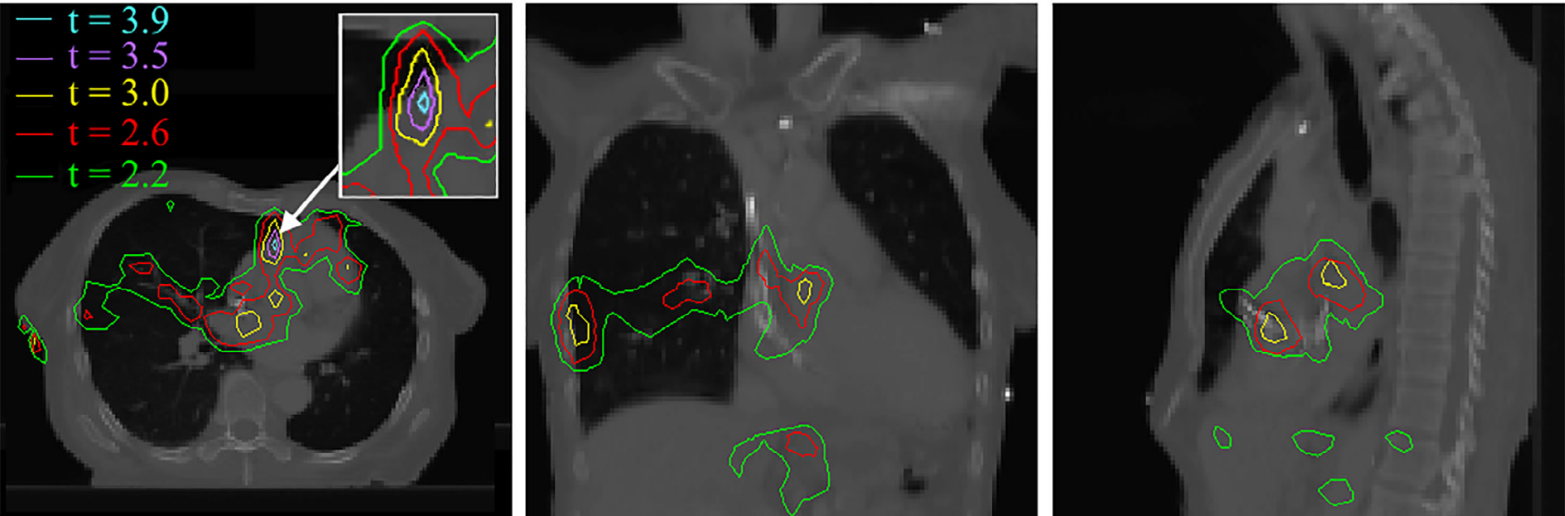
Data mining results of Δdose vs survival at one year. Axial, coronal and sagittal views of the reference patient CT, with the *t*-values overlaid, where the *t*-value provides a measure of the statistical significance of the region over the permutation testing. The most significant region is highlighted by the arrow. Patients who did not survive one year had a significantly higher Δdose in this region.
